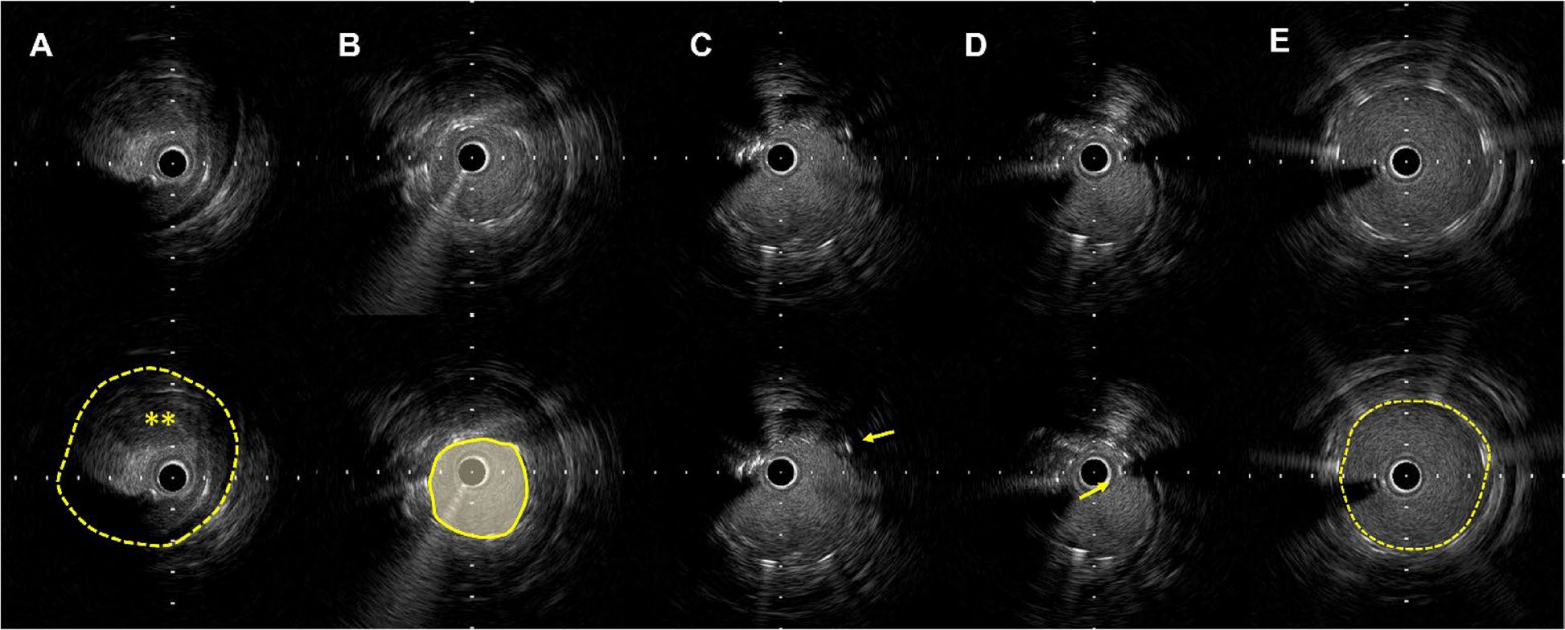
IVUS image. A Baseline IVUS findings: thrombus present (). B Post-invaginated stent findings: insufficient dilation (3.4 × 2.9 mm). C Wire cross from outside stent strut (yellow arrow). D Check wire crossings in the stent. E IVUS after treatment, confirming sufficient dilation (5.2 × 5.1 mm)IVUS, intravascular ultrasound
